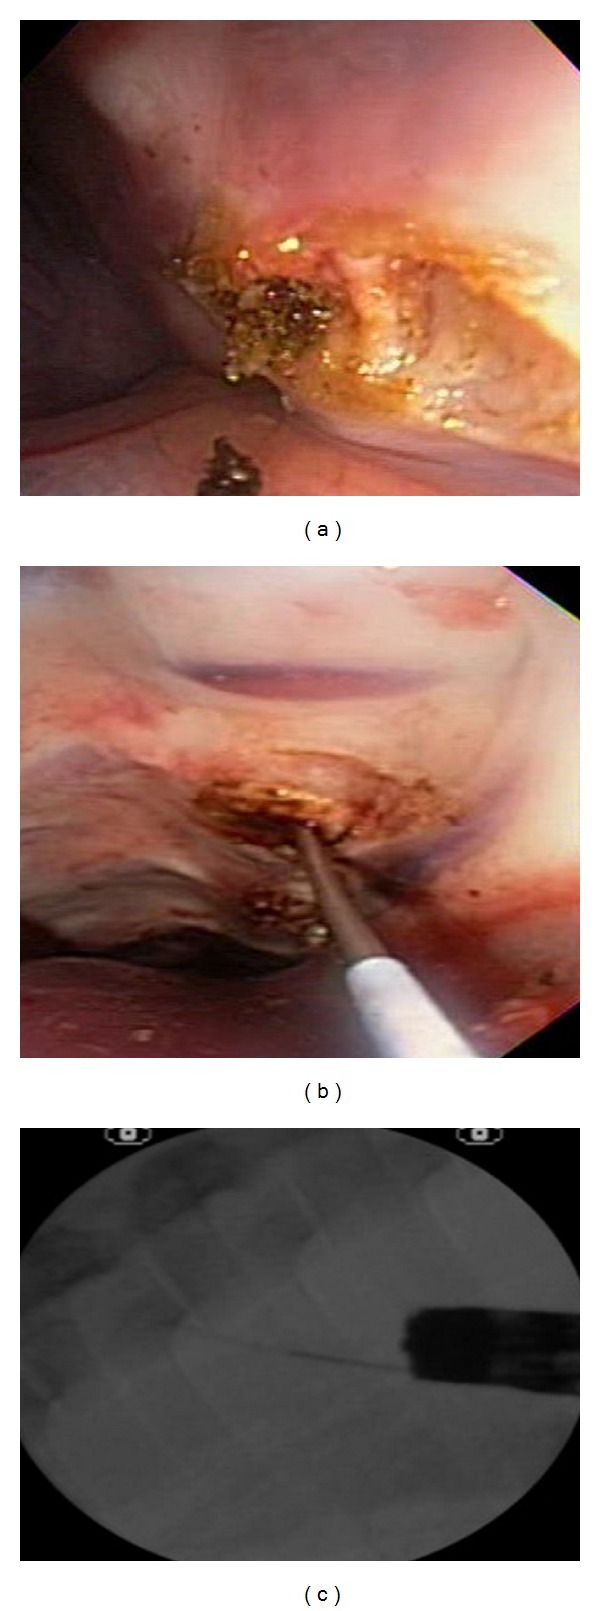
Transesophageal approach to the anterior thoracic spine. (a) Incision over the anterior longitudinal ligament and exposure of the intravertebral space and vertebral bone. (b) Insertion of the 19 gauge needle in the thoracic vertebrae. (c) Fluoroscopic view of vertebral bone biopsy.
